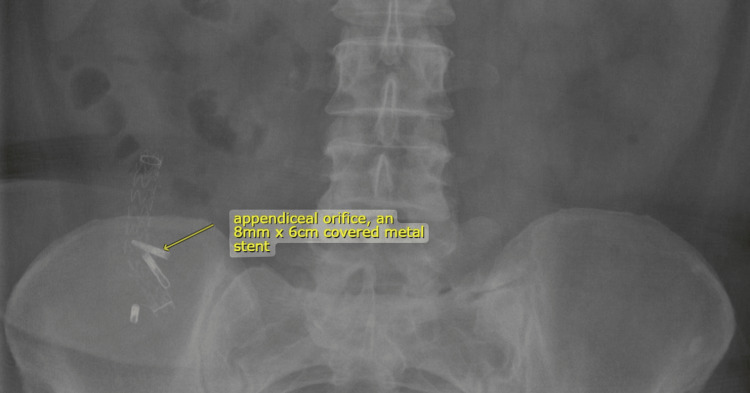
X-ray of the abdomen demonstrating appendiceal stent
